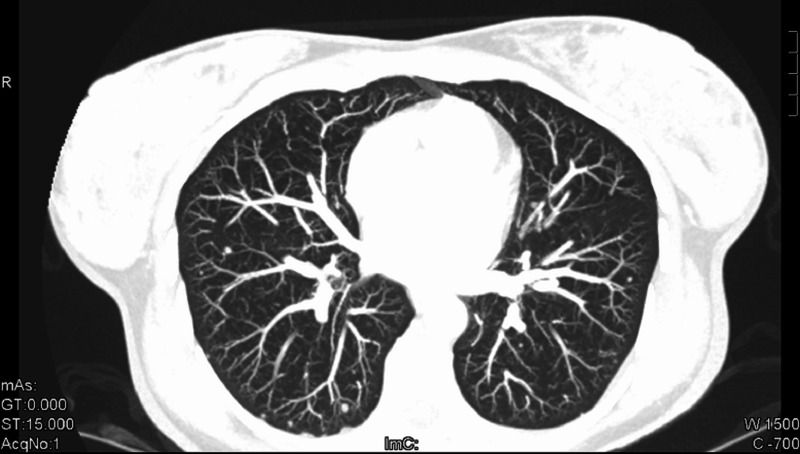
Patient 2: CT imaging demonstrates lung nodules pre treatment
